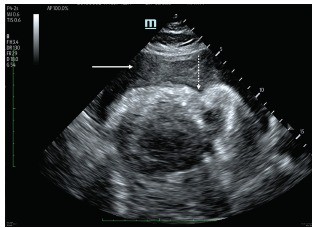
Subxiphoid cardiac ultrasound demonstrating a large complex pericardial effusion (solid arrow) with complete right ventricular (RV) collapse and bowing of the RV free wall (dashed arrow).
